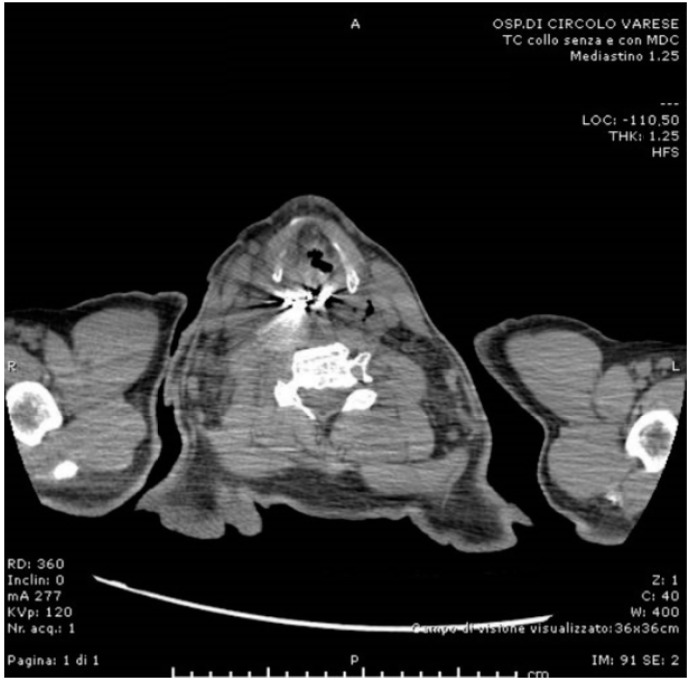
Preoperative CT scan (sagittal).
